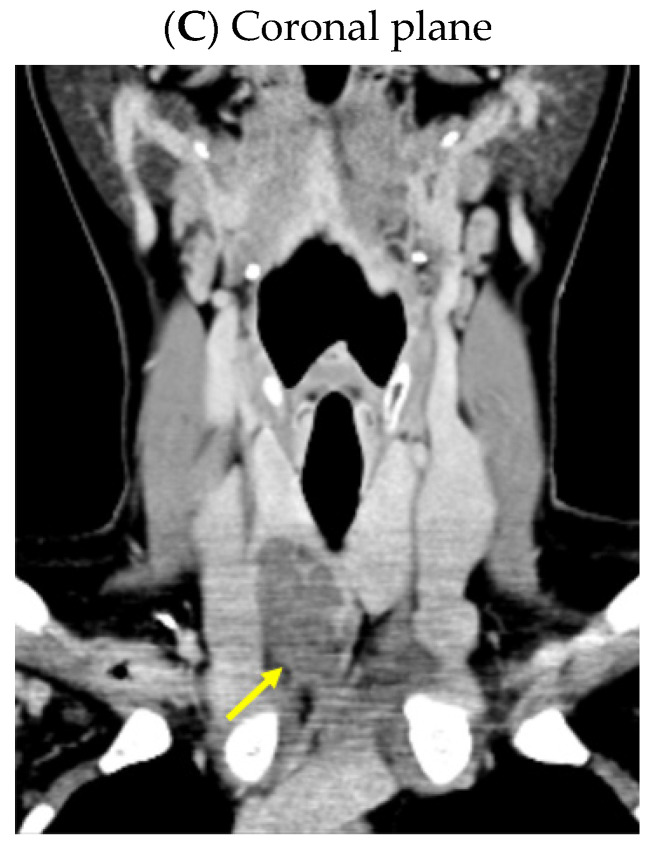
Intravenous contrast CT scan: right inferior parathyroid tumor (yellow arrow) displayed as a hypodense nodule of 3.98 by 1.13 by 2.53 cm.
